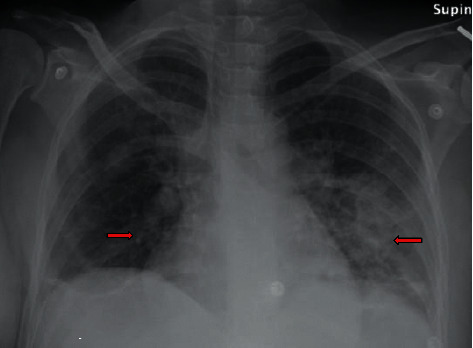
Chest X-ray revealed diffuse bilateral lower lobe predominant airspace opacities with nodularity.
